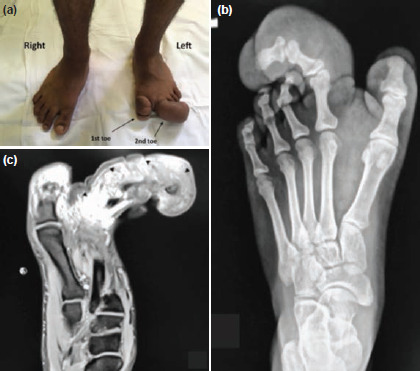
Clinical and radiological features of MDL (a) Clinical features of overgrowth of left 1st and 2nd toe. (b) Radiograph of MDL showing a laterally deviated 2nd toe with enlarged phalanges and soft tissue overgrowth. (c) Sagittal view of T1 weighted MRI of the foot showing excess subcutaneous tissue of the 2nd toe (arrowhead).
